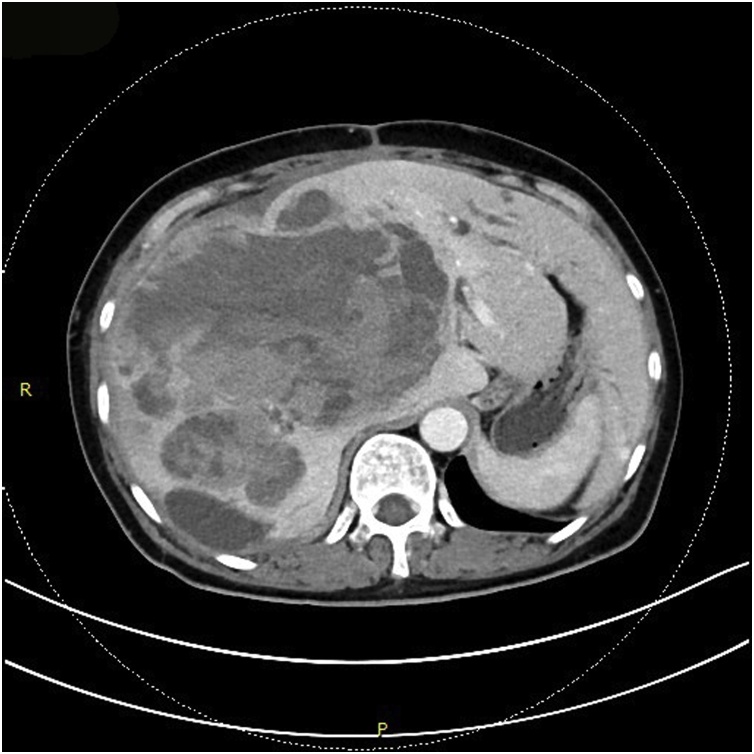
CT scan showing recurrence of hepatic multiple cysts.
